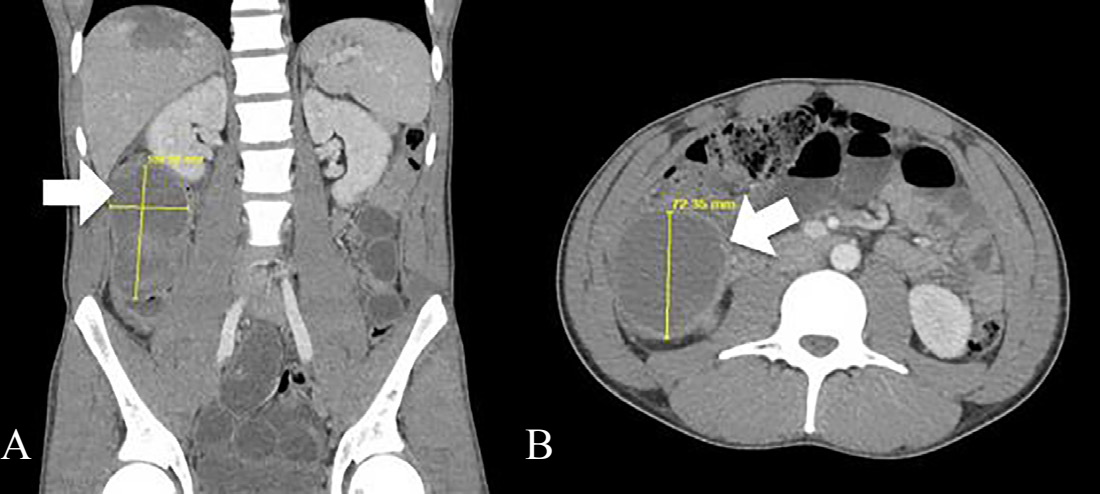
Computed tomography with contrast, showing a large (10.1×5.9×7.2 centimeters), heterogeneous intraluminal collection of fluid in the ascending colon (arrows). A. Coronal view. B. Axial view.
